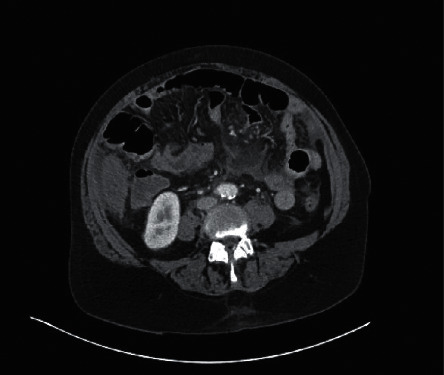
Initial computed tomography (CT) of the abdomen and pelvis with small bowel enteritis and fluid collections in paracolic gutters.
